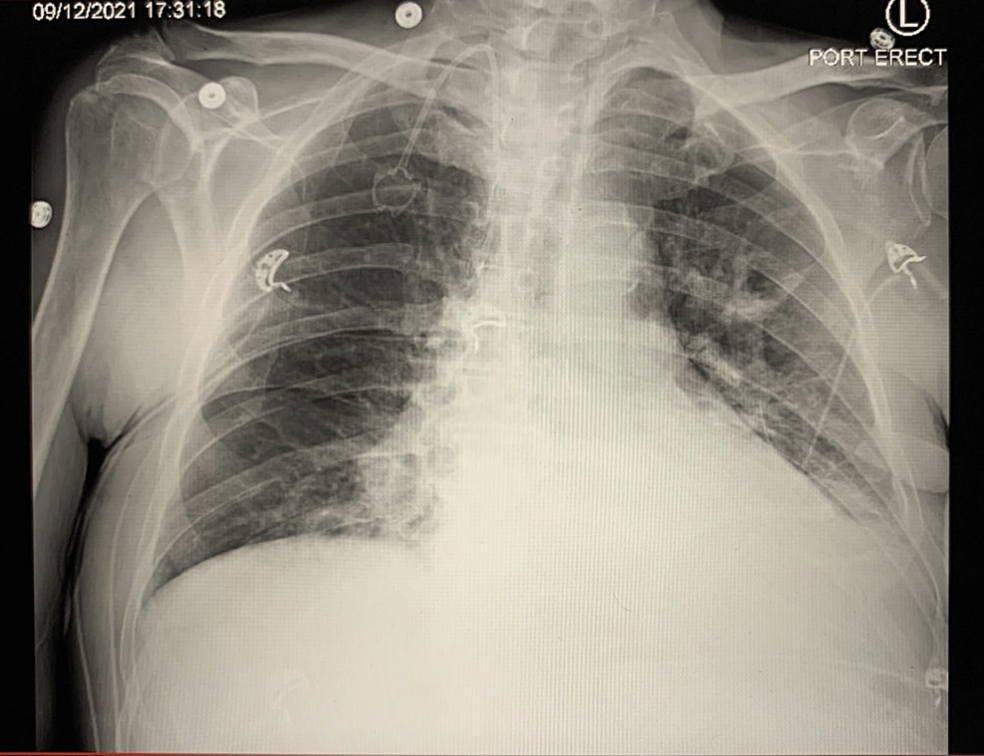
Chest X-ray showing left lower lobe infiltrates on day two of admission
